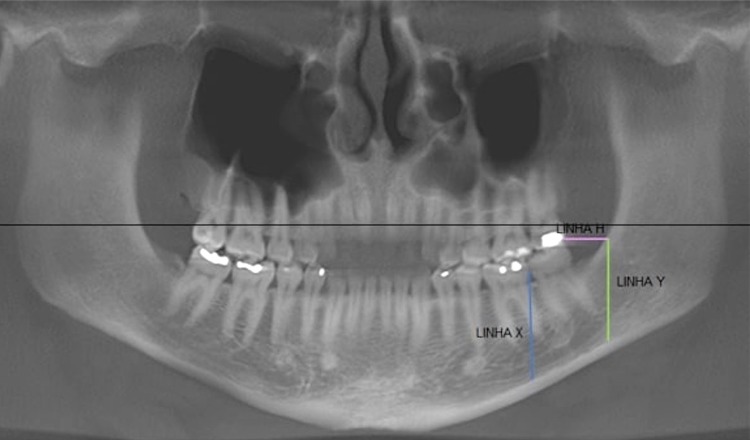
CBCT panoramic reformation showing the positions of Lines X, H and Y, which represent the limits of interest in the evaluation
